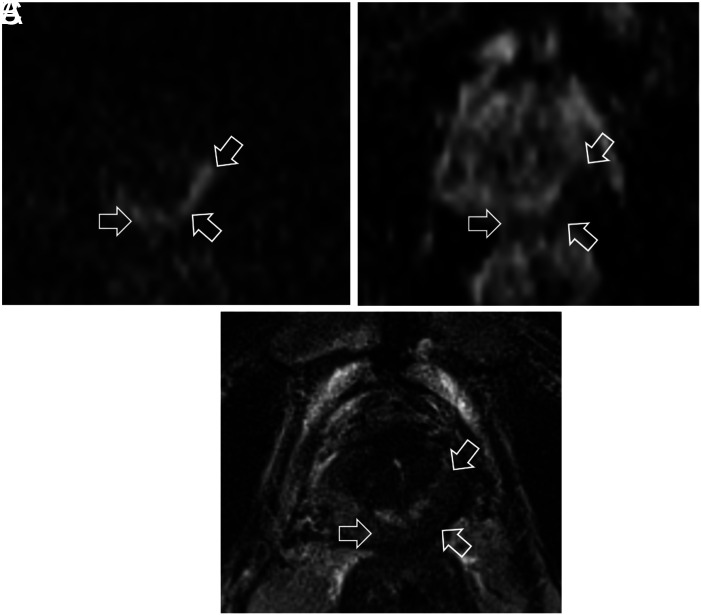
PI-RRADS category 4 based on biparametric MRI, 2 years after RT for prostate adenocarcinoma Gleason score 8, in 75-year-old patient with increased PSA 2.4 ng/mL The lesion detected as hyperintense on DWI at high *b* values (arrow in A) and markedly hypointense on ADC map (arrow in B) and hypointense inhomogeneous on axial T2W imaging (arrow in C) occurs at the site of the tumor of origin in the mid-gland in the left lateral segment of the peripheral zone.
